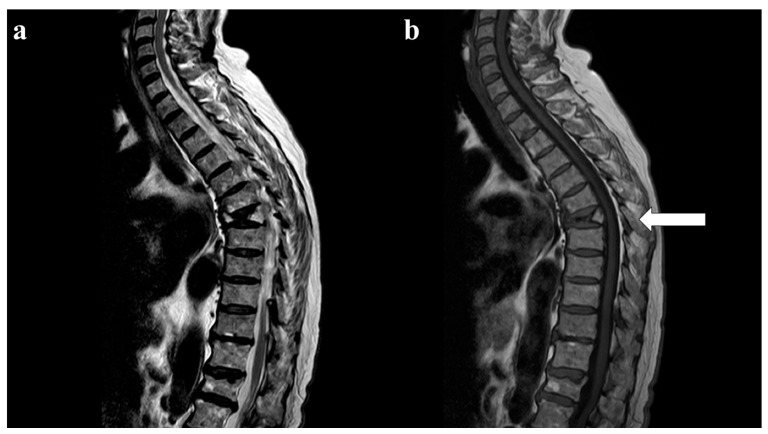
Post-traumatic vertebra plana in a 75-year-old female. (a) Sagittal T2 and (b) T1 weighted MRI show acute wedge fractures of T7 without the involvement of the posterior wall and medullary canal (white arrow).
